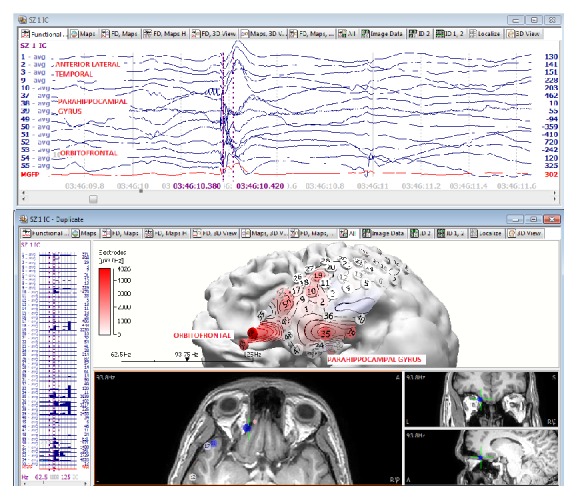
HFO mapping of initial ictal activity at the orbitofrontal and mesial temporal ictal activity of a typical seizure. The EEG data on top depicts the time window where the HFO power was calculated. The bottom image on the left side displays the graphic bar power on each EEG channel and the right side represents the localization onto a 3D model based on the patient's own MRI.
